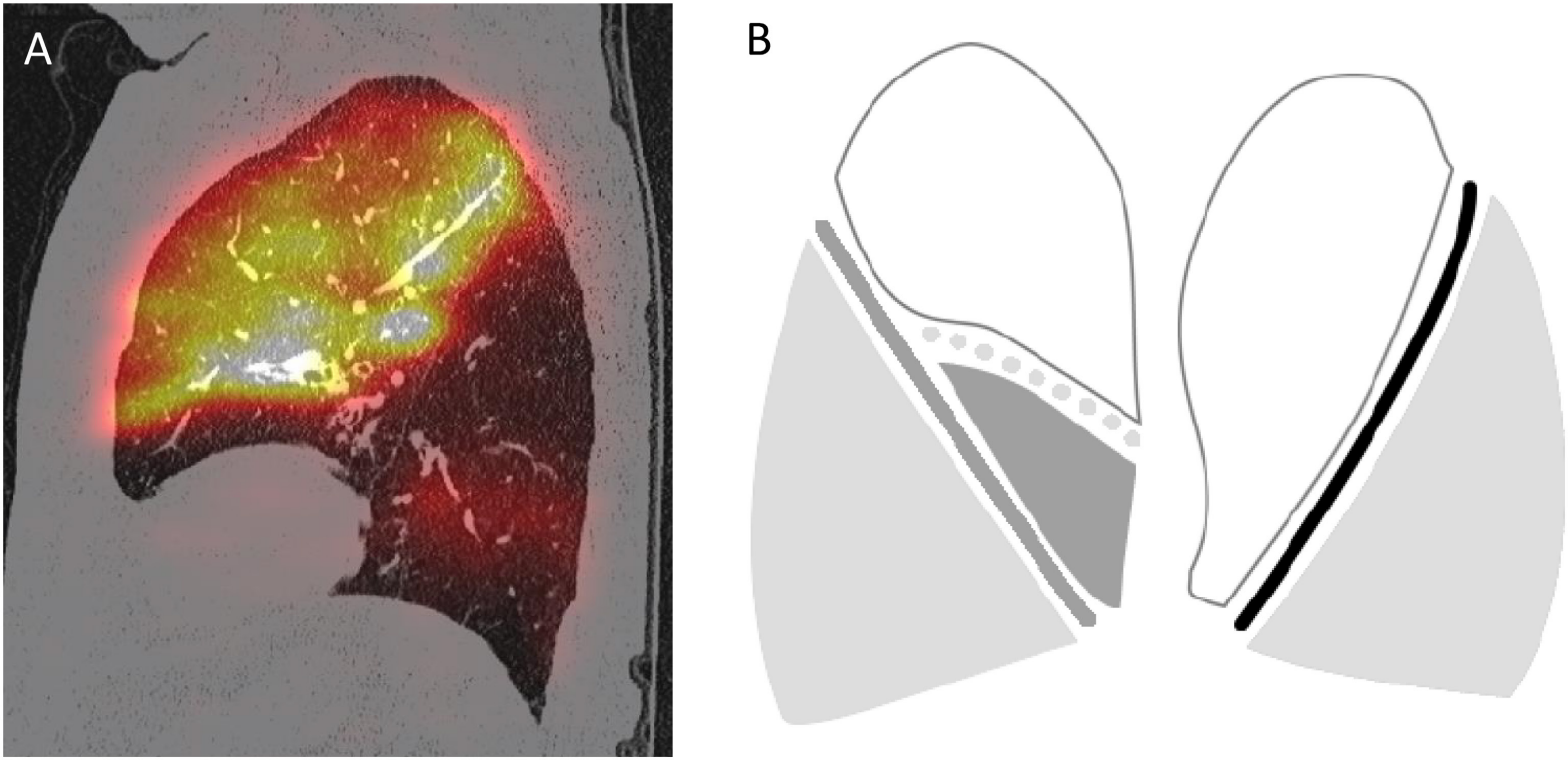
(A) Quantitative lung analysis performed using StratX Lung Analysis Platform (Pulmonx Corporation, Redwood City, CA, USA). (B) Sagittal view of lung perfusion scintigraphy obtained using single‐photon emission computed tomography (SPECT) (OptimaTM CT540, GE Healthcare, Chicago, IL, USA).
